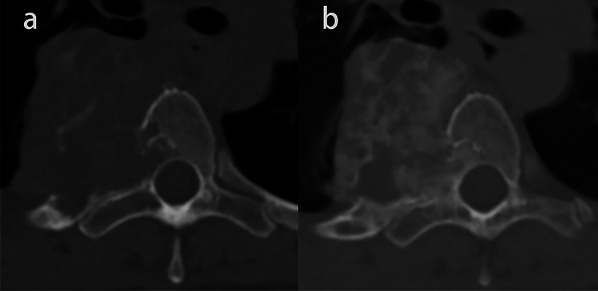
Giant cell tumor of T4 in a 40-year-old woman. a Axial CT scan before denosumab treatment. b Axial CT scan after denosumab treatment shows a complete peripheral rim and more than 50% of the transverse session ossified (grade 3)
